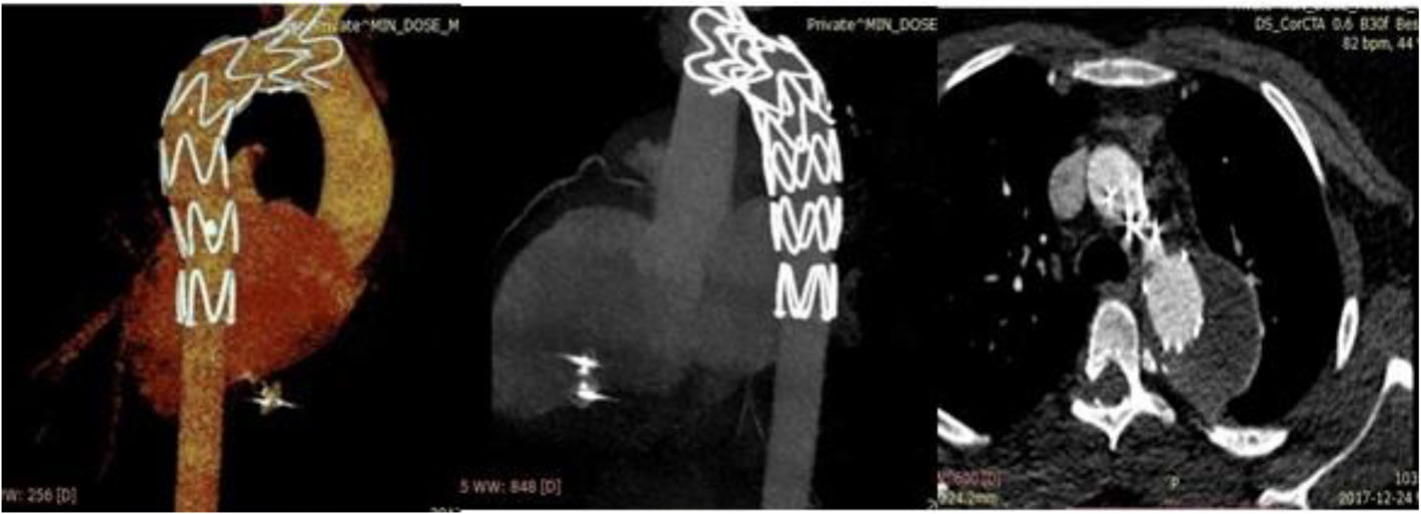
Follow-up CT angiography showing complete exclusion of the aneurysm
